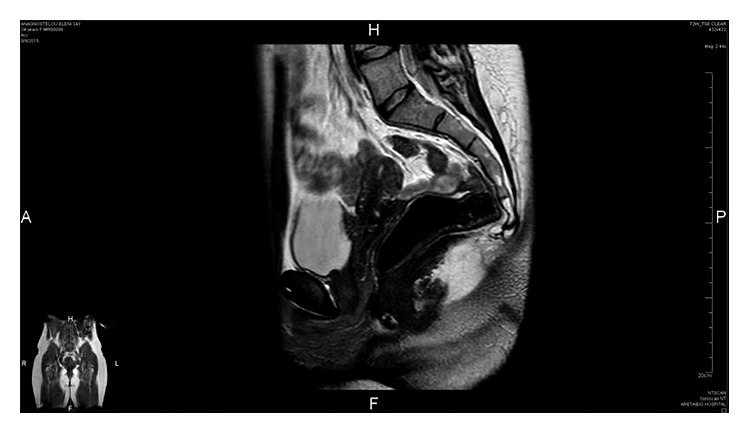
Magnetic resonance image showing a case of deep endometriosis infiltrating the dome of the bladder.
